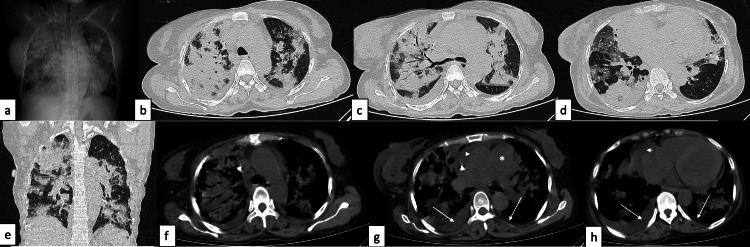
Fungal infection - Aspergillus fumigatus A 45-year-old female patient with SLE presented with complaints of fever, cough, chest pain, and shortness of breath of 22-day duration. (a) The topogram image showed extensive areas of consolidation in bilateral lung fields. Axial (b-d) and coronal (e) HRCT chest images in the lung window confirmed extensive areas of consolidation in both lungs with ground-glass opacities in the right middle lobe. (f-h) Additionally, a dilated main pulmonary artery (g, asterisk), cardiomegaly with mild pericardial effusion (arrowheads), and bilateral mild pleural effusions (white arrows) were observed in the mediastinal window. Features were suggestive of infective aetiology, likely bacterial. Culture from bronchoalveolar lavage confirmed fungal infection (Aspergillus fumigatus). SLE: systemic lupus erythematosus; HRCT: high-resolution CT
